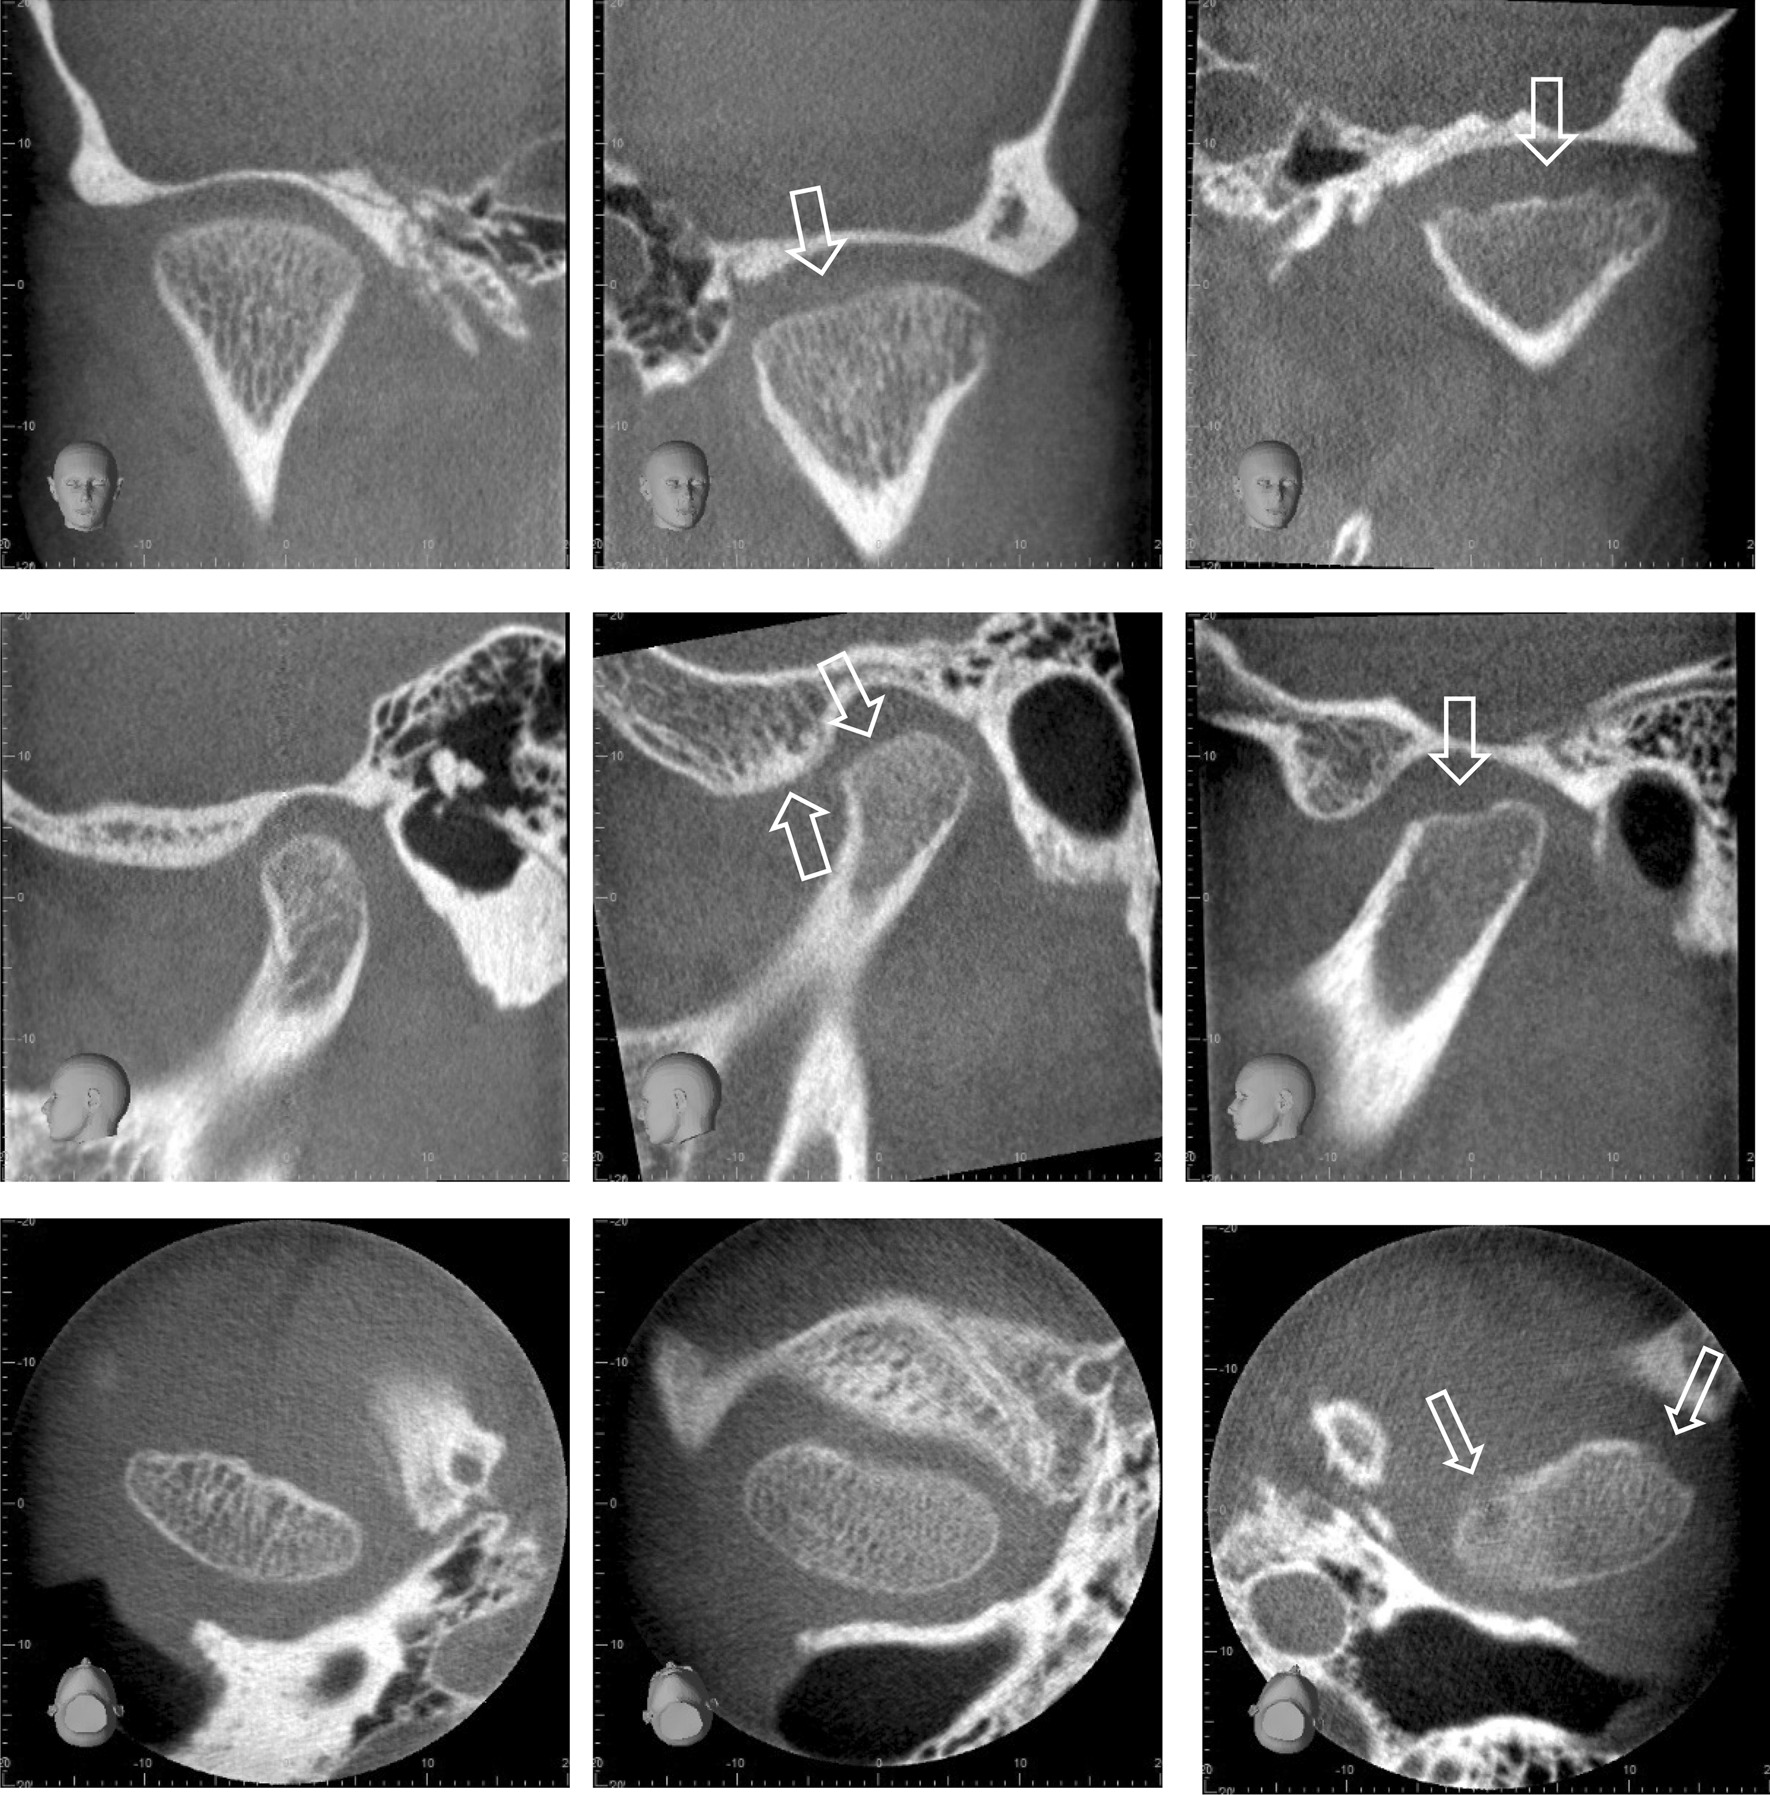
Examples of temporomandibular joint (TMJ) deformity on cone-beam computed tomography (CBCT)
